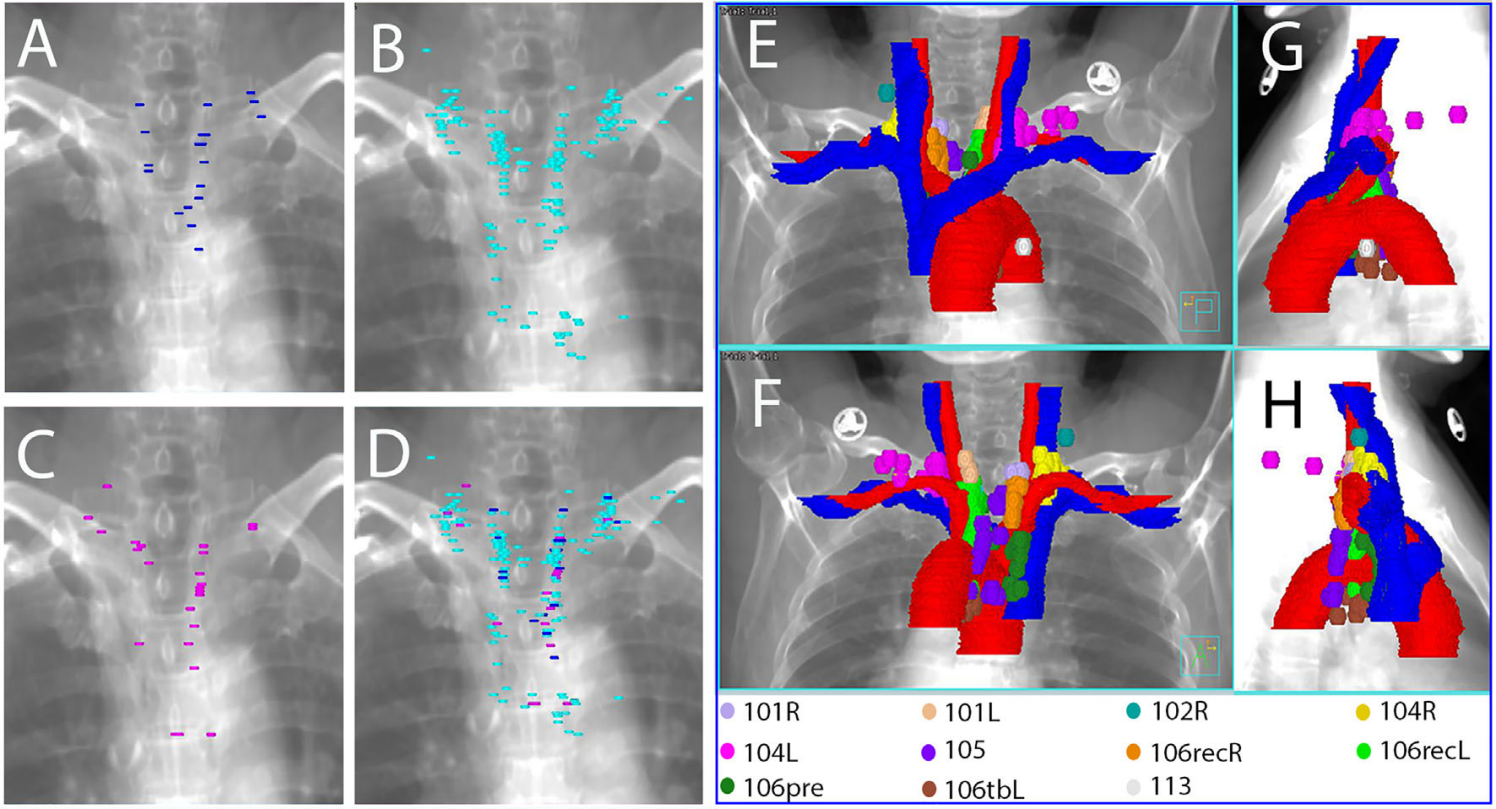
Reconstructed images with all of the plotted LNs (A–D) and all of the TVnds (E–H) on the template CT. (A) anterior-posterior viewpoint for upper TESCCs; (B) anterior-posterior viewpoint for middle TESCCs; (C) anterior-posterior viewpoint for lower TESCCs; (D) anterior-posterior viewpoint for all TESCCs; (E) anterior-posterior viewpoint for all TESCC; (F) posterior-anterior viewpoint for all TESCCs; (G) right-left viewpoint for all TESCCs; (H) left-right viewpoint for all TESCCs.
